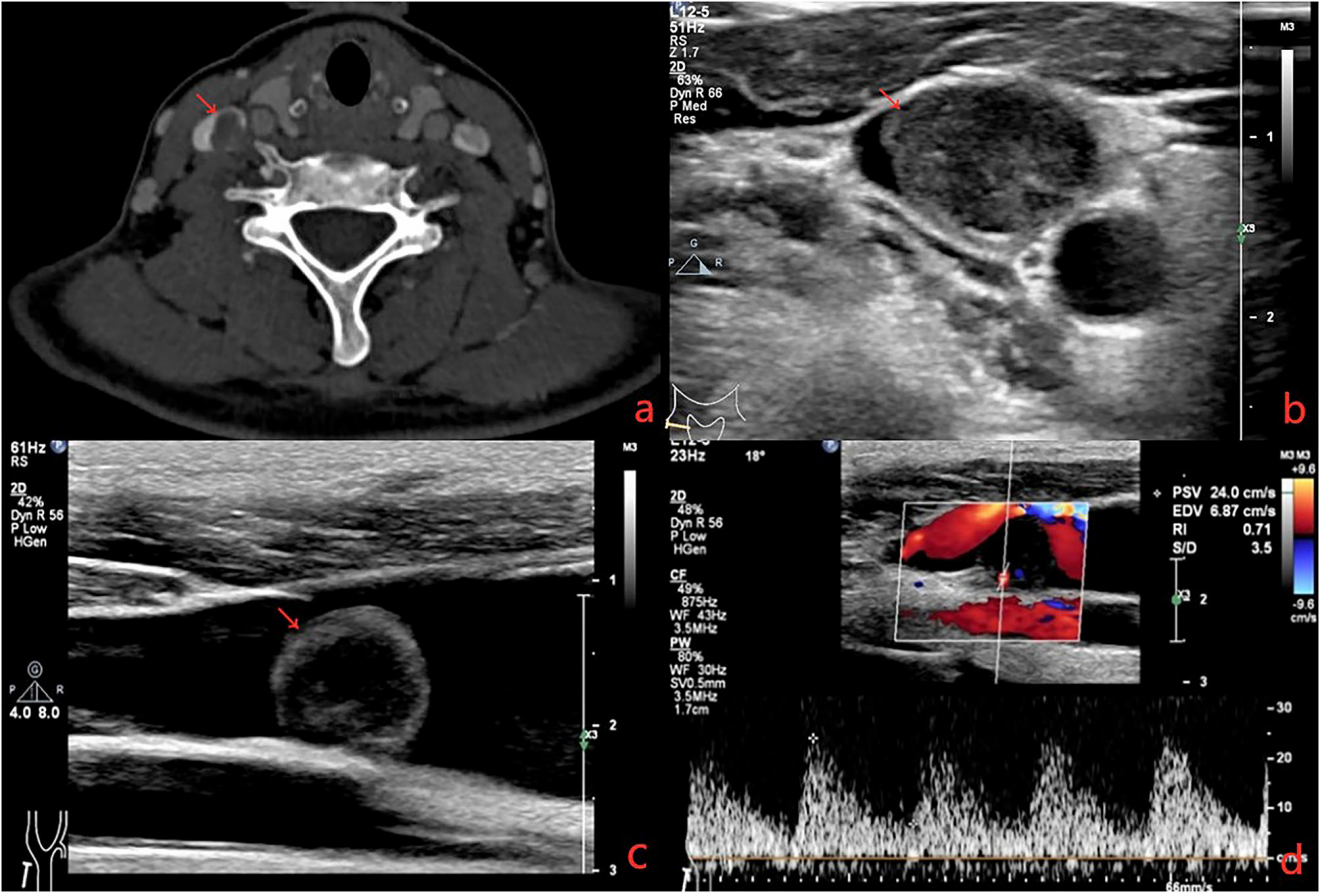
(a) Computerized tomography shows a solid, non-enhanced nodule in the right internal jugular vein (RJV) (red arrow: nodule, green arrow: RJV). (b) Ultrasound shows the nodule arising from the RJV without extravascular invasion (red arrow: nodule, green arrow: RJV). (c) Ultrasound shows the nodule led to severe RJV stenosis (red arrow: nodule, green arrow: RJV, red triangle: right internal carotid artery). (d) Doppler ultrasound detected the blood flow signals in the nodule.
